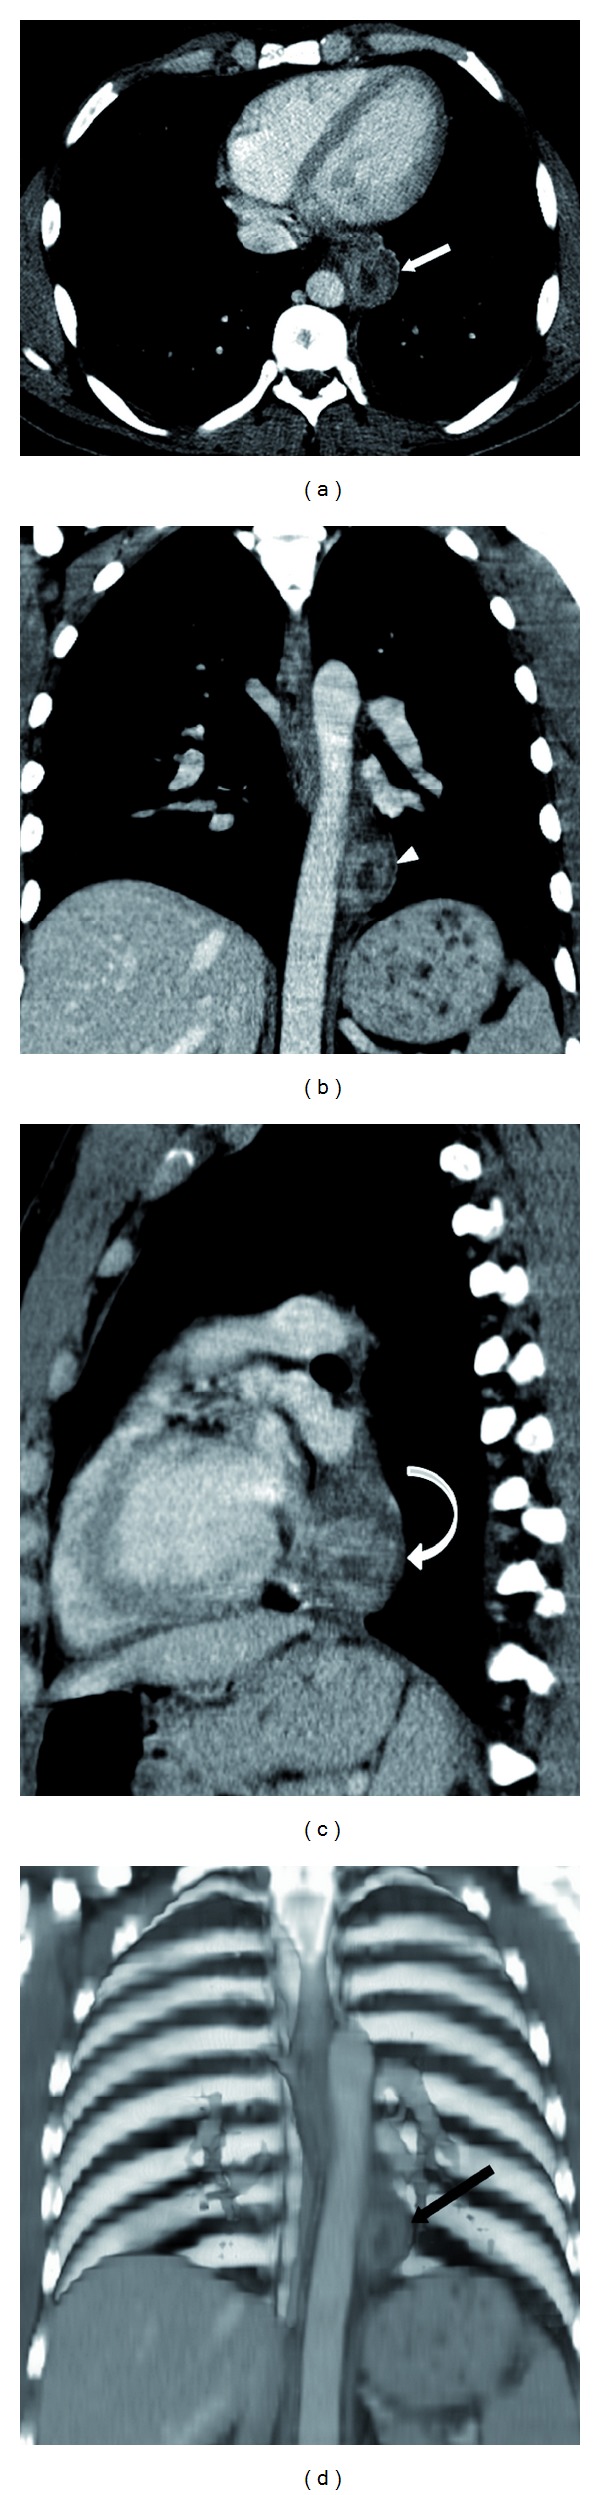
(a) Contrast enhanced CT axial image showing the presence of a rounded mass with central hypoattenuation adjacent to the esophagus (arrow). Images obtained on a Siemens Somatom Sensation 64 slice after administration of intravenous contrast (Isovue 370) 100 mL. Total DLP 1128.81 mGycm. (b) Coronal multiplanar reformatted CT image showing the mass adjacent to the thoracic aorta (arrowhead). (c) Sagittal multiplanar reformatted CT image showing the retrocardiac mass (curved arrow). (d) Volume rendered coronal reformatted CT image showing the central hypoattenuation within the paraesophageal mass (black arrow).
